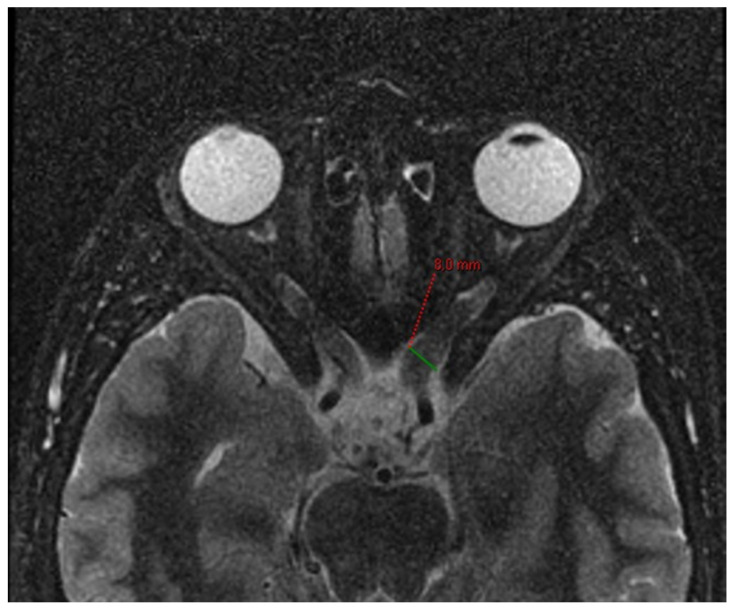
Optic nerve glioma in a patient affected by NF1. Axial T2-weighted FSE with fat suppression. The pre-chiasmatic tract of the left optic nerve has a diameter of approximately 8 mm (green line).
